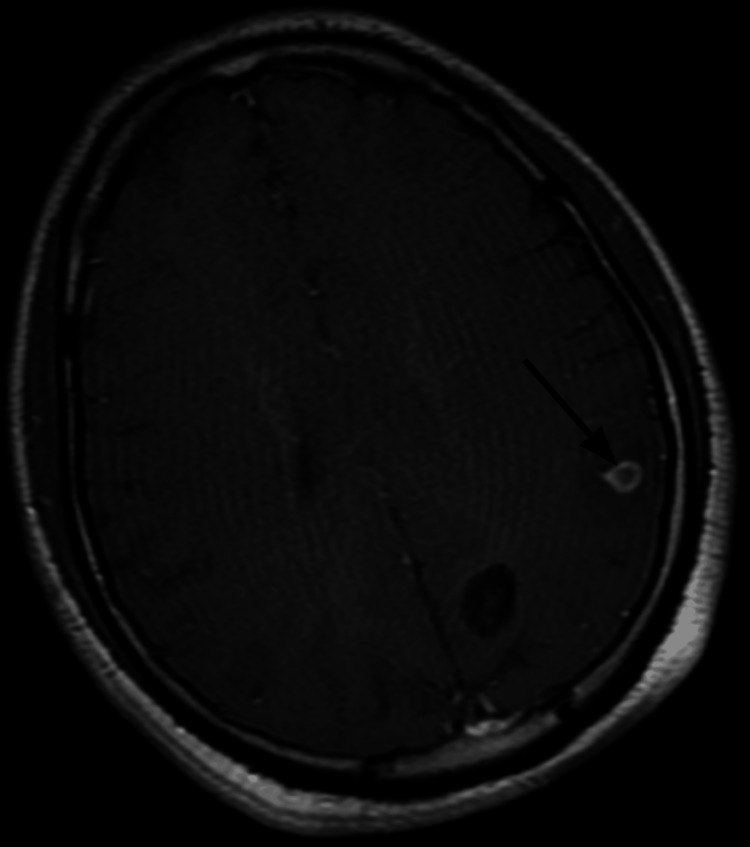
MRI of the brain T1 sequence confirms the presence of a cystic lesion in the left parietal lobe
